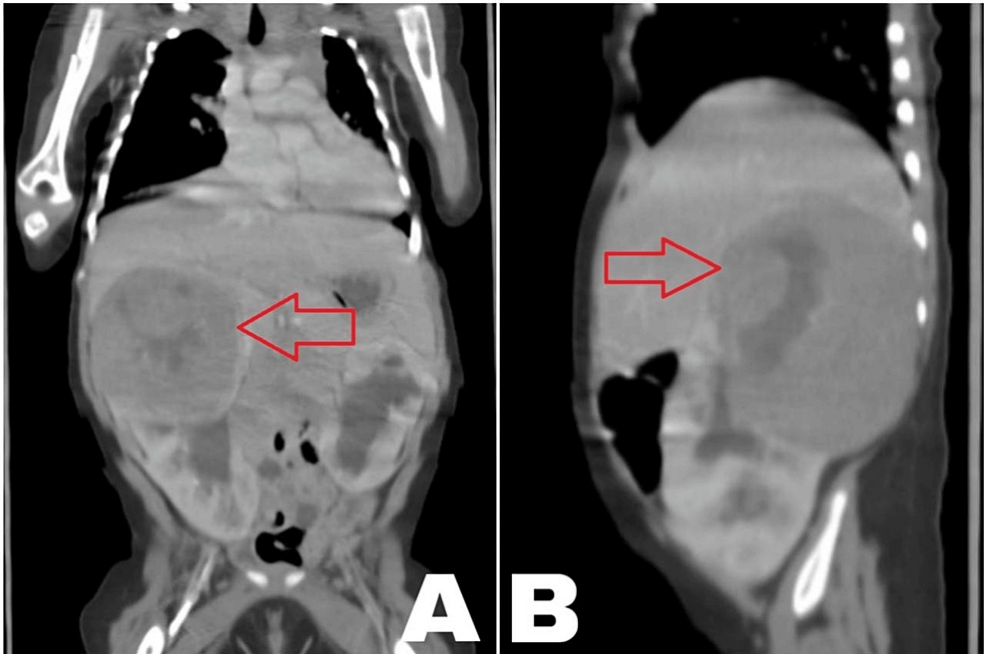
CT scan of the abdomen Coronal view (A): shows a large, poorly enhancing mass with central necrosis arising from the upper pole of the right kidney. Please note the bilateral moderate hydronephrosis. Sagittal view (B): The upper pole of the right kidney is largely replaced by the mass with a bulging contour causing a mass effect upon the right hepatic lobe.
